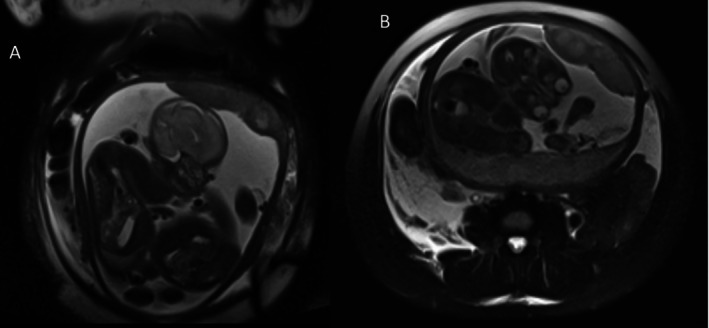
Coronal (A) and Axial (B) T2W MRI images showing a gravid uterus with twins and moderate free peritoneal collection.
